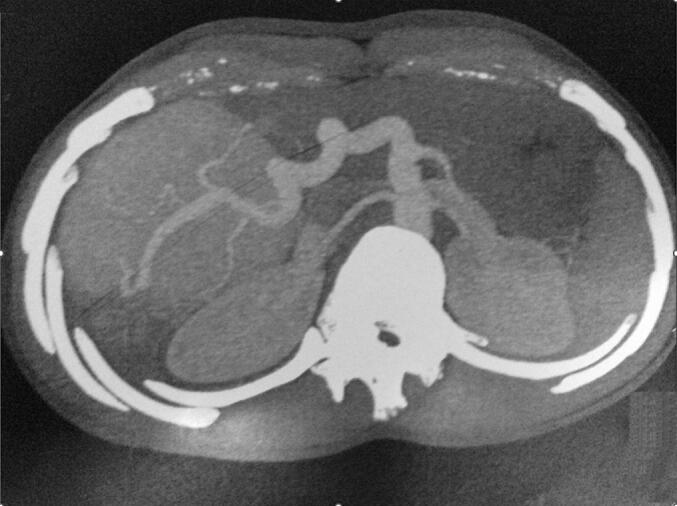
A contrast enhanced CT (CECT) scan: a large mixed density mass in the right lobe of liver with presence of a focal hypodense area in the center of the lesion.
